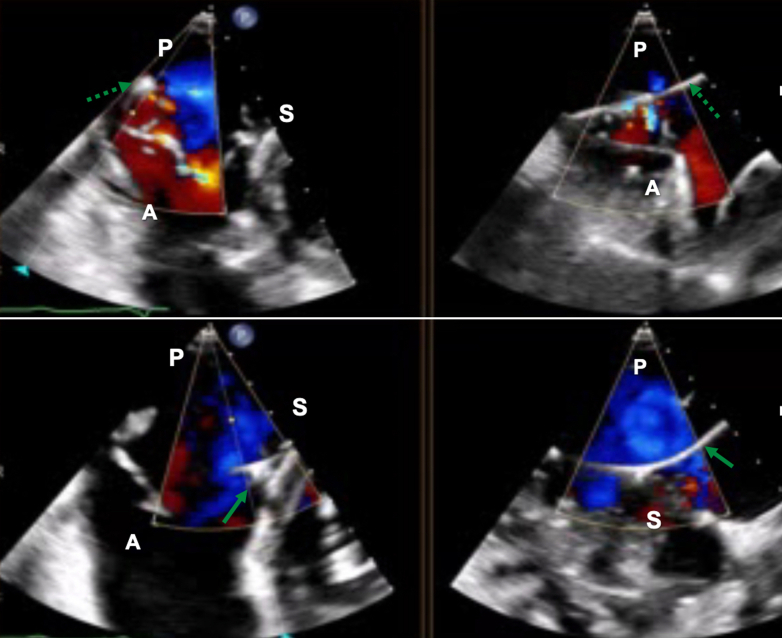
Transesophageal Echocardiographic Assessment of Tricuspid Regurgitation Transesophageal echocardiography showing biplane transgastric en face images of the tricuspid valve, focusing on both the anteroposterior (upper panel) and anteroseptal commissure (lower panel), with evidence of septal leaflet impingement by one of the 2 right ventricular leads (solid green arrow) and posterior leaflet perforation by the other lead (dashed green arrow). A = anterior; P = posterior; S = septal.
